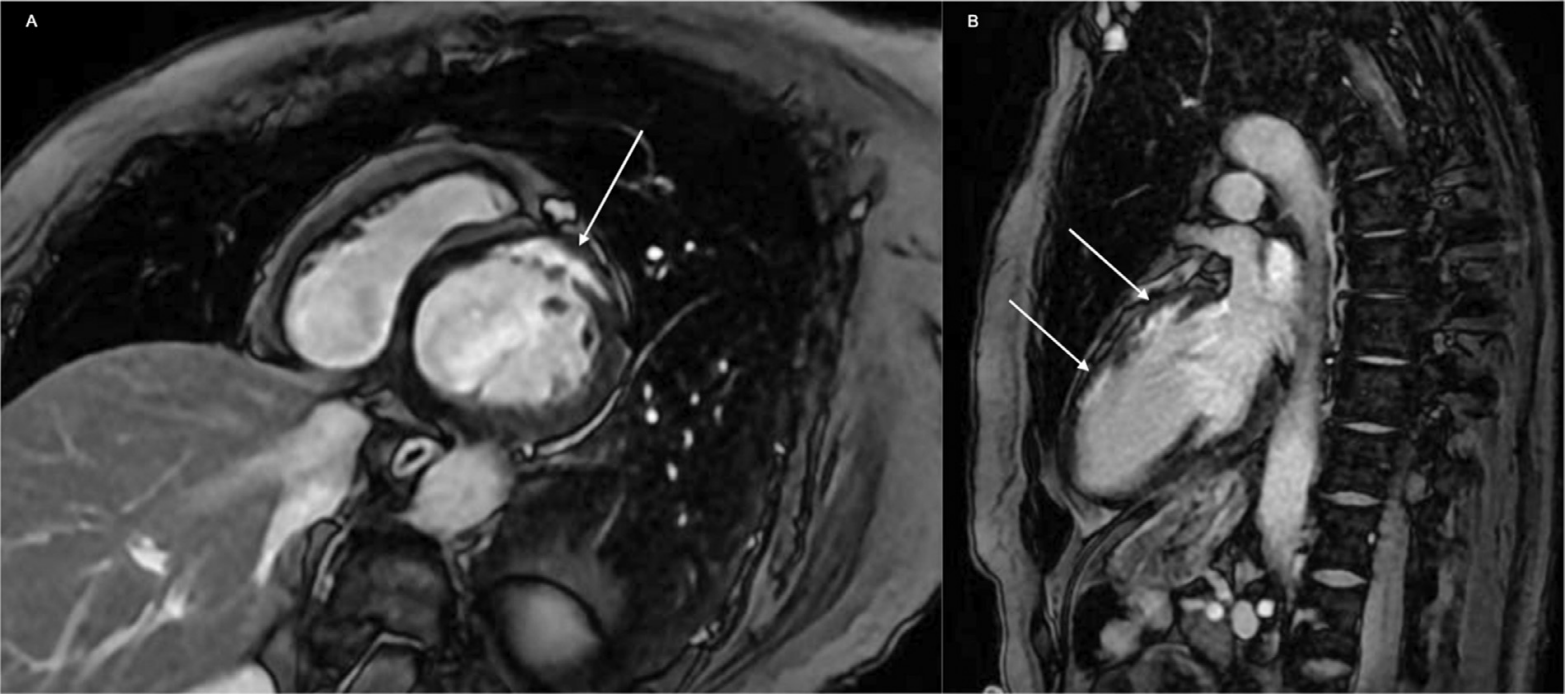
Cardiac MRI short axis (A) and 2-chamber (B) images of a bSSFP (balanced steady-state free precession) sequence acquired 10 minutes after contrast agent injection. The white arrows show the area of subendocardial late enhancement, involving the anterior wall of the left ventricle with a typical ischemic pattern.
